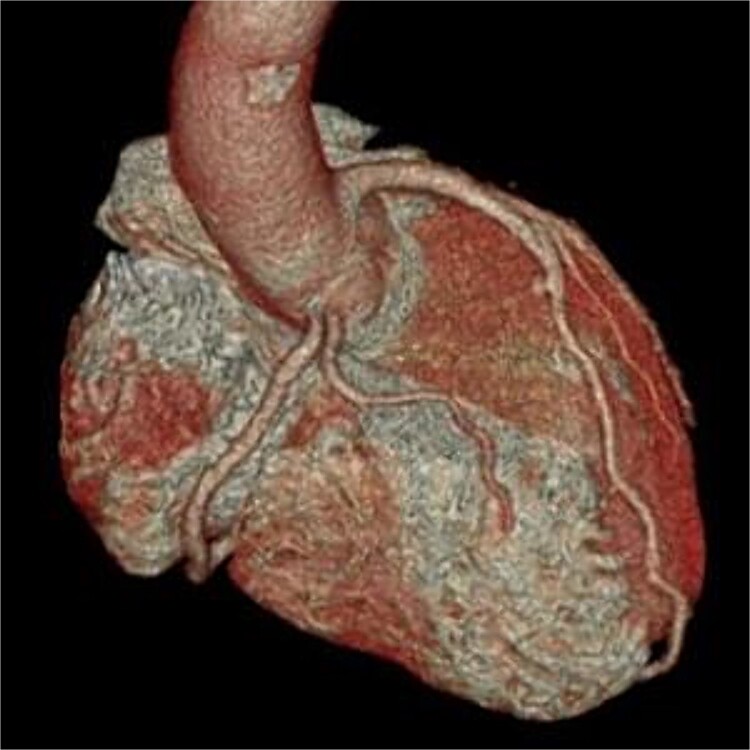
Postoperative three-dimensional CT, and enhanced CT shows the patency of the right coronary artery.
